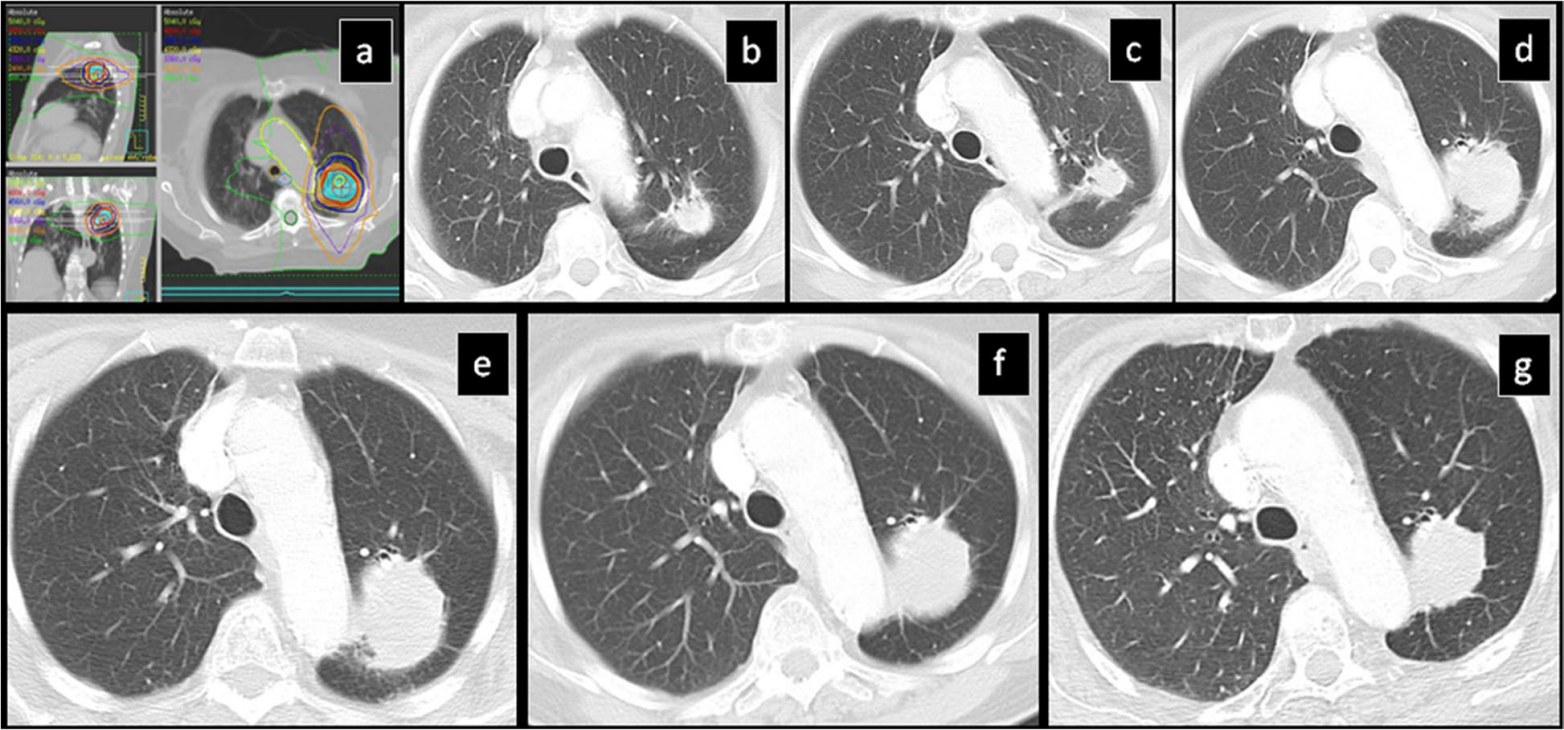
SBRT-treated lung cancer continues to decrease in size at 49 months: a) Isodose multiplanar images for planning. b) pre-SBRT CT shows a solid nodule. c) 6 mo post-SBRT, the primary lesion slightly decreases in size and there is mild adjacent peribronchial consolidation. d) 9 mo post-SBRT, the lesion significantly increases in overall size with mild surrounding peribronchial consolidation. e) 18 mo post-SBRT, the lesion size is unchanged and there is improvement of the surrounding peribronchial consolidation, f) 22 mo post-SBRT, there is mild decrease of the lesion size and resolution of surrounding peribronchial consolidation, g) 49 mo post-SBRT, there is further mild decrease in lesion size
